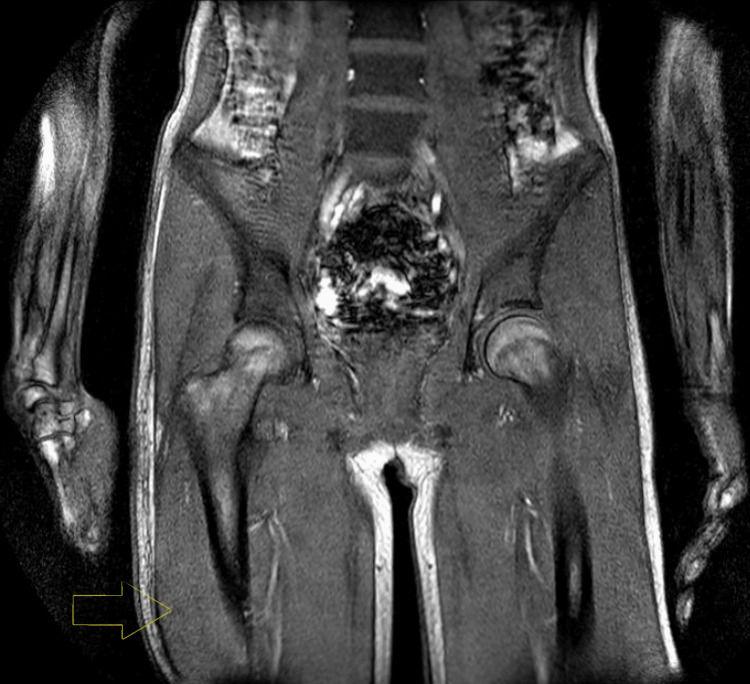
MRI of the lower body showing extensive bilateral involvement of the thigh musculature. Features of dermatomyositis are indicated by an arrow. MRI, magnetic resonance imaging
